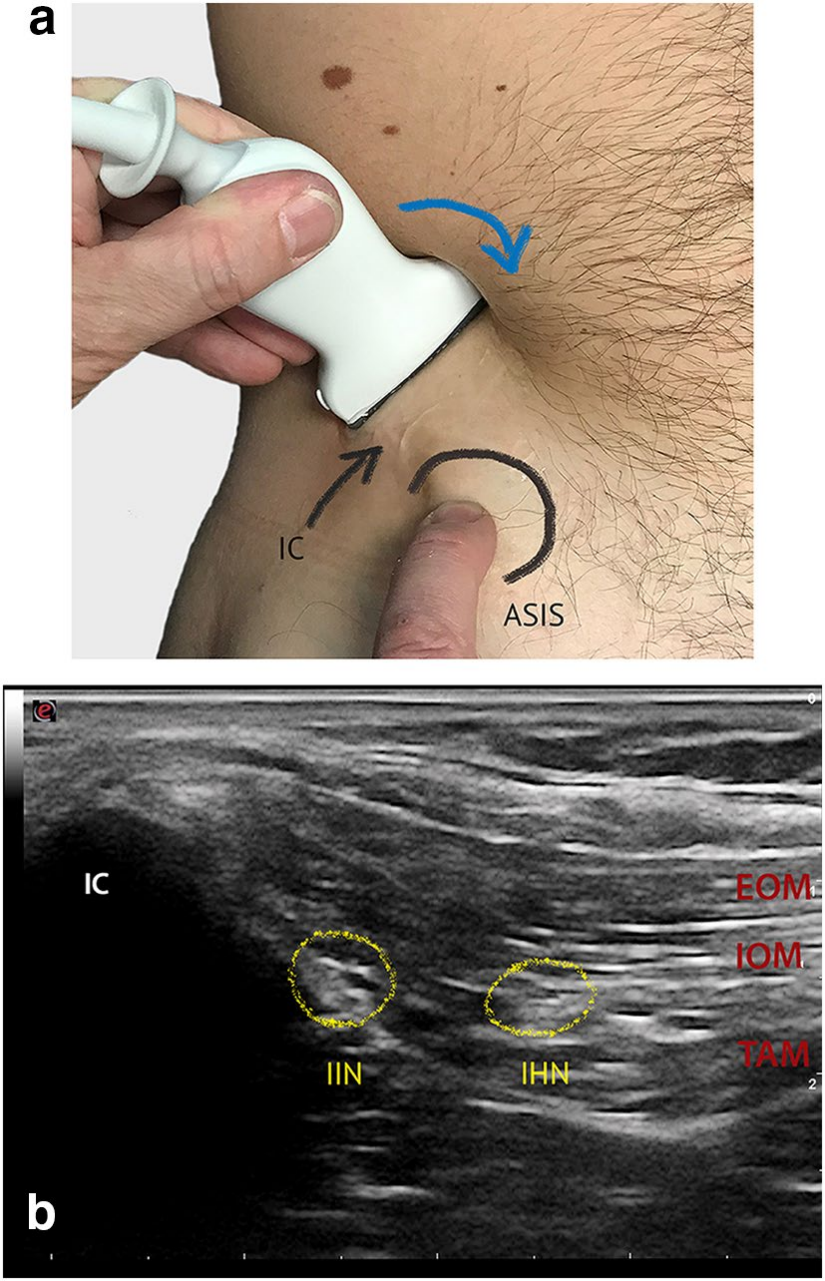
Probe placement for high-resolution ultrasonographic visualization of IHN and I-IN (ESAOTE, Italy, 13 MHz, linear array probe). a The arrow indicates the probe movement. The ASIS (black circle) and IC as landmarks. b Ultrasonographic image of I-IN and IHN obtained by the probe position. I-IN and IHN (yellow circles) lying in between the Internal oblique (IOM) and Transversal abdominal muscle (TAM). *IC* iliac crest
